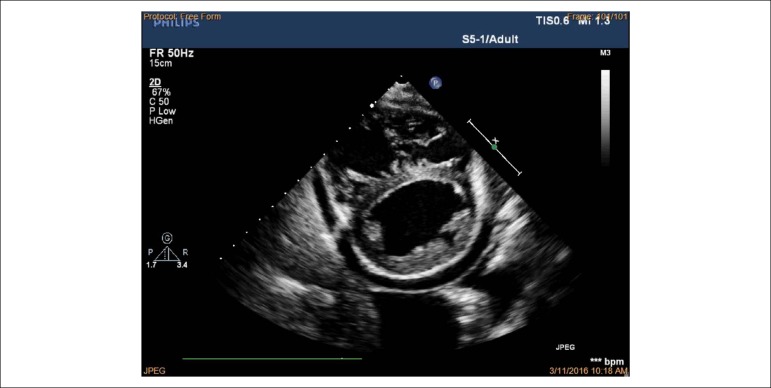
Transthoracic echocardiography from the parastrenal short axis view
